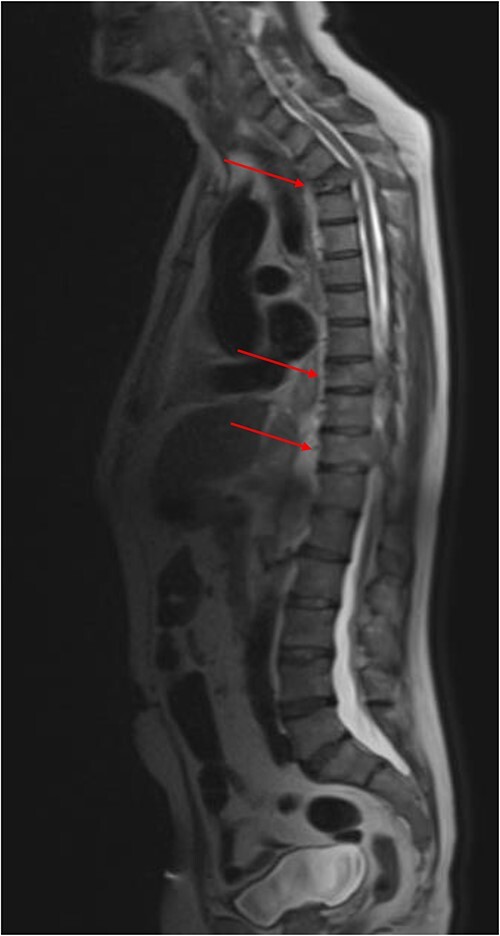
Sagittal MRI whole-spine demonstrating extensive bony metastatic disease. Malignant compromise to the spinal canal at T3, T9 and T11 (red arrows).
